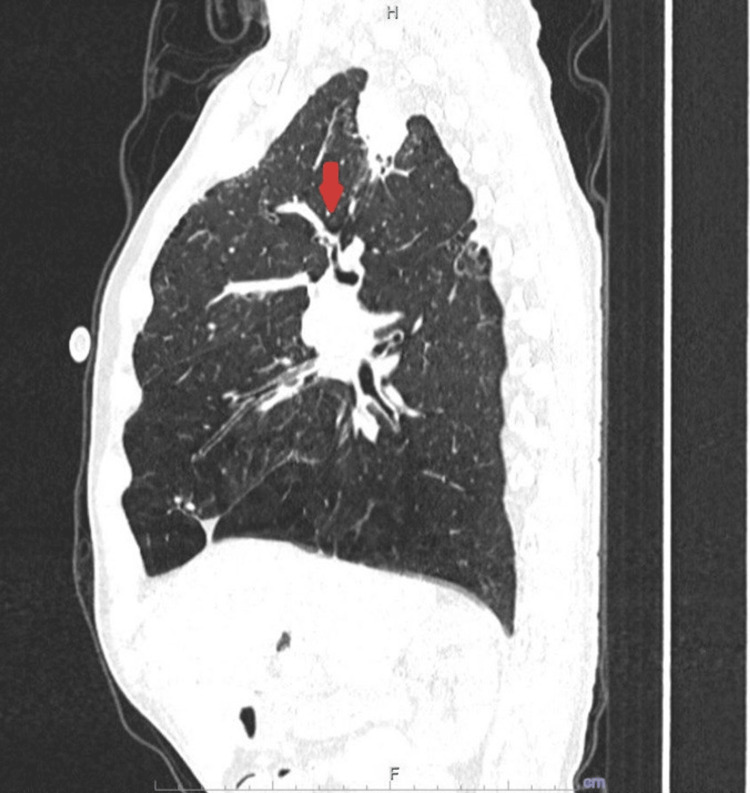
CT of the thorax (coronal): bilateral upper lobe opacities with cavitation (red arrow)
